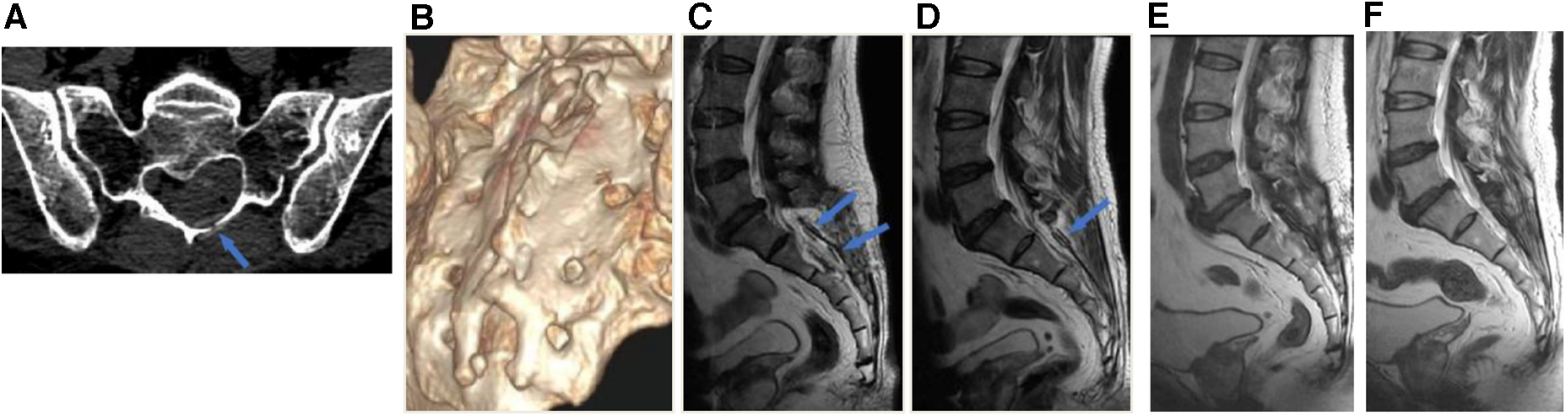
Postoperative radiological assessments. (A) The absorbable clamp (arrow) can be seen at the left side of the laminotomy on the postoperative axial CT image. (B) Postoperative 3D-CT showed sacral laminoplasty with excellent planarity and stability. (C) Postoperative 2-week MRI T2-weighted image showed 2 absorbable carniofixes at the right side of sacral laminectomy (blue arrows). (D) Postoperative 2-week MRI T2-weighted image showed 1 absorbable carniofix at the left side of sacral laminectomy (blue arrow). (E and F) Postoperative 6-month MRI revealed the bone structure of the sacral canal posterior wall remained stable, in comparison to the MRI at 2 weeks post-surgery, there was a significant improvement in the reduction of soft tissue swelling in the para-vertebral region.
